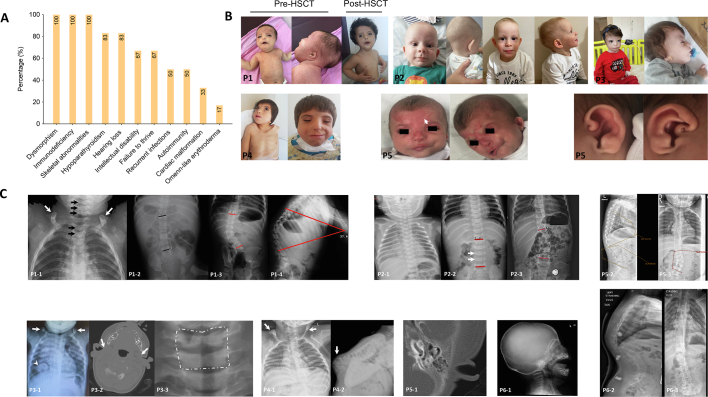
Clinical phenotypes of PAX1-deficient patients showing specific features. (A) The main manifestations of PAX1 deficiency. (B) Representative pictures of patients' phenotypes: (P1) Facial dysmorphisms (bilateral microtia, nasal root flattening, hypertelorism, epicanthus, and micrognathia), alopecia, and Omenn-like generalized rash (resolved with HSCT). (P2) Dysmorphisms characterized by low-set ears, short nose with low nasal bridge, hypertelorism, epicanthus, micrognathia, long philtrum, and thin upper lip. (P3) Micro- and retrognathia, maxillary hypoplasia, telecanthus, bilateral epicanthus, small and anteverted nostrils, microtia, low set ears, down slanting palpebral fissures, and thin upper lip. (P4) Facial dysmorphism including hypertelorism, flattened nasal root, epicanthus, flat philtrum, long eyelashes, right facial paralysis, bilateral preauricular cupping, microtia, short neck, and microdontia. (P5) Low-set ears with pre-auricular pits, overfolded ears, and a hypoplastic mandible with retrognathia. Flat nasal bridge with nevus flammeus (arrow) / asymmetric face. (C) Radiological findings of the patients. P1–1: Chest X-ray with no thymus shadow, butterfly (C4-T1) and split (T2-T4) vertebral bodies (black arrows), and hooked distal clavicles (white arrows). P1–2 and P1–3: Increased lumber dextroscoliosis centralized at the level of L1 over time (Cobb angles: 5.2^°^ (black) *vs.* 33.3^°^ (red)). P1–4: Thoracolumbar kyphosis. P2–1: A rudimentary thymus shadow on chest X-ray. P2–2: Hypoplastic vertebrae (L4-L5) on X-ray spin (white arrows). P2–2 and P2–3: Increased lumber levoscoliosis centralized at the level of L2 over time (Cobb angles (red lines): 1.8^°^*vs.* 18.8^°^). P3–1: Chest X-ray shows hooked distal clavicles (arrows) and multiple nodular opacities with pneumonic infiltration (arrow head). P3–2: Temporal bone computed tomography demonstrates bilateral external auditory canal atresia (white arrows). P3–3: C6 and C7 butterfly vertebrae on X-ray graph (white square). P4–1: Hooked distal clavicles (arrows). P4–2: Gibbus on chest X-ray graph (white arrows). P5–1: Aplastic or fully obstructed external auditory meatus, with no eardrum visible. Hypoplastic ossicles and increased sclerosis in the cochlear and semicircular canal region. P5–2 and P5–3: Hyperkyphosis and lumbar levoscoliosis. P6–1: Prominent mid-face with beak-like appearance. P6–2 and P6–3: Scoliosis on X-ray of the whole spine. HSCT: Hematopietic stem cell transplantation. (For interpretation of the references to color in this figure legend, the reader is referred to the web version of this article.)
